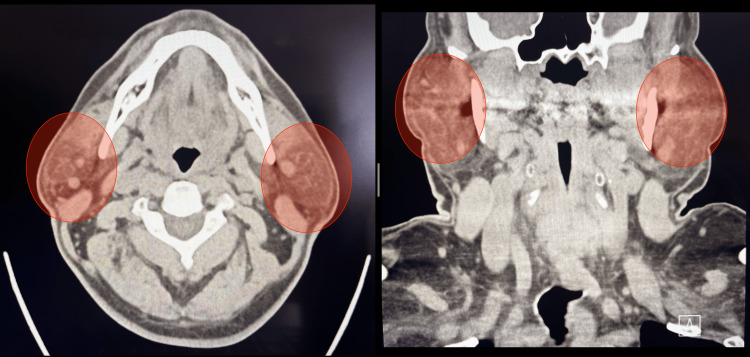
Axial and coronal planes of CT scan showing bilateral parotid swelling (highlighted in red).
